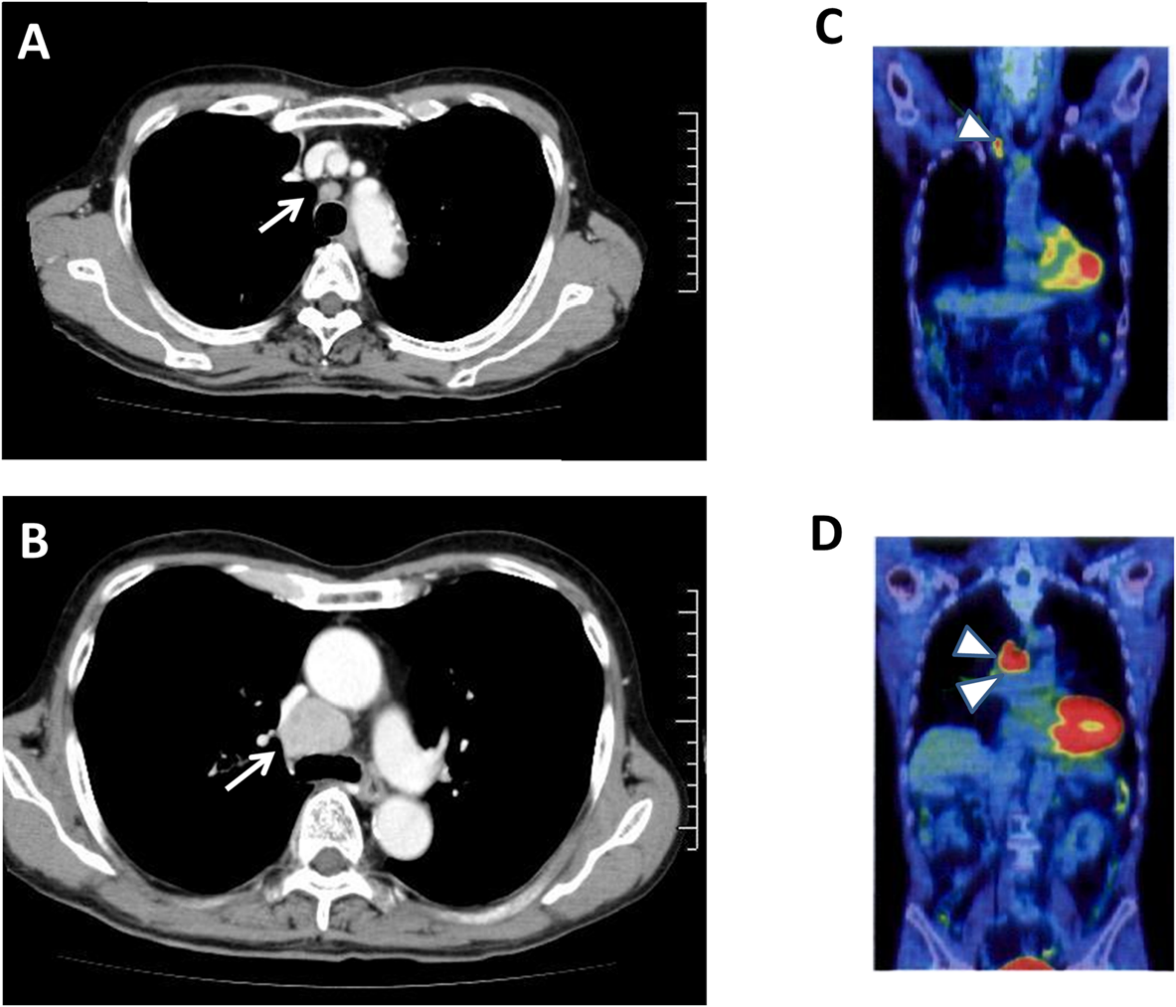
Enhanced computed tomography revealed swelling of the right upper paratracheal lymph node (a, *arrow*) and right lower paratracheal lymph node (b, *arrow*). Abnormally high uptake in the right supraclavicular lymph node (c, *arrowhead*) and right lower paratracheal lymph node (d, *arrowheads*) was detected by positron emission tomography/computed tomography. The right lower paratracheal lymph node was the main lesion, which was approximately 2.7 cm in size. The right upper paratracheal lymph node and right supraclavicular lymph node were metastatic lesions and showed slight swelling
